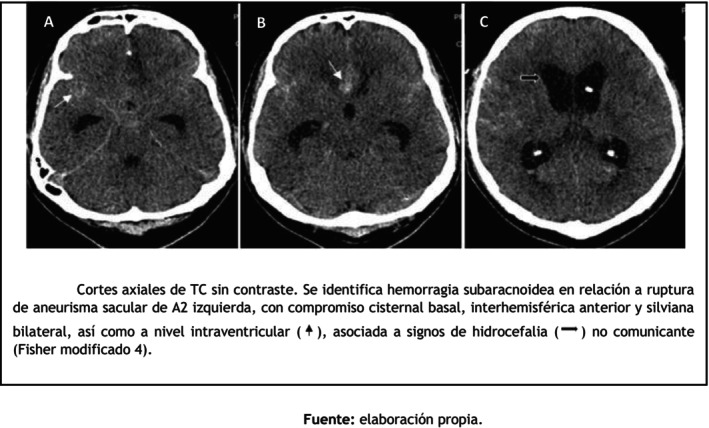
Non‐contrast axial brain CT images demonstrating subarachnoid hemorrhage secondary to rupture of a left A2 saccular aneurysm, with blood distribution involving the basal cisterns, anterior interhemispheric fissure, bilateral sylvian fissures, and intraventricular extension (
